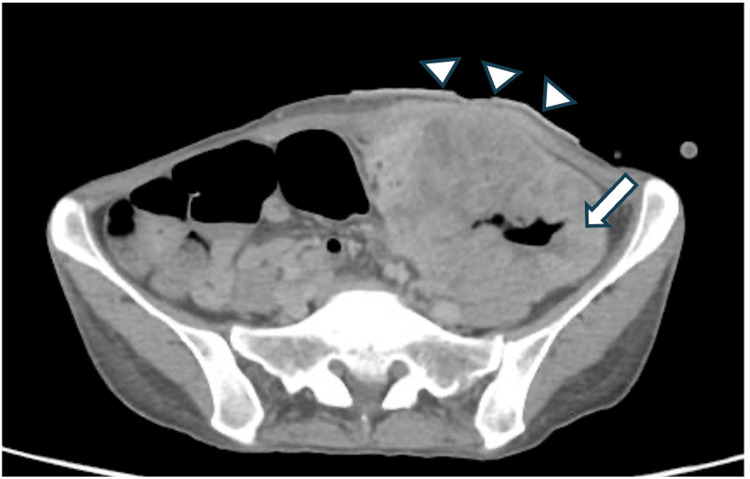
CT findings at the initial visit CT at the time of diagnosis reveals an approximately 9.7 cm tumor in a single mass in the sigmoid colon (arrow), with encapsulated air and fluid collection in the surrounding area and abscess formation. The tumor invades the subcutaneous tissues (arrowheads).
